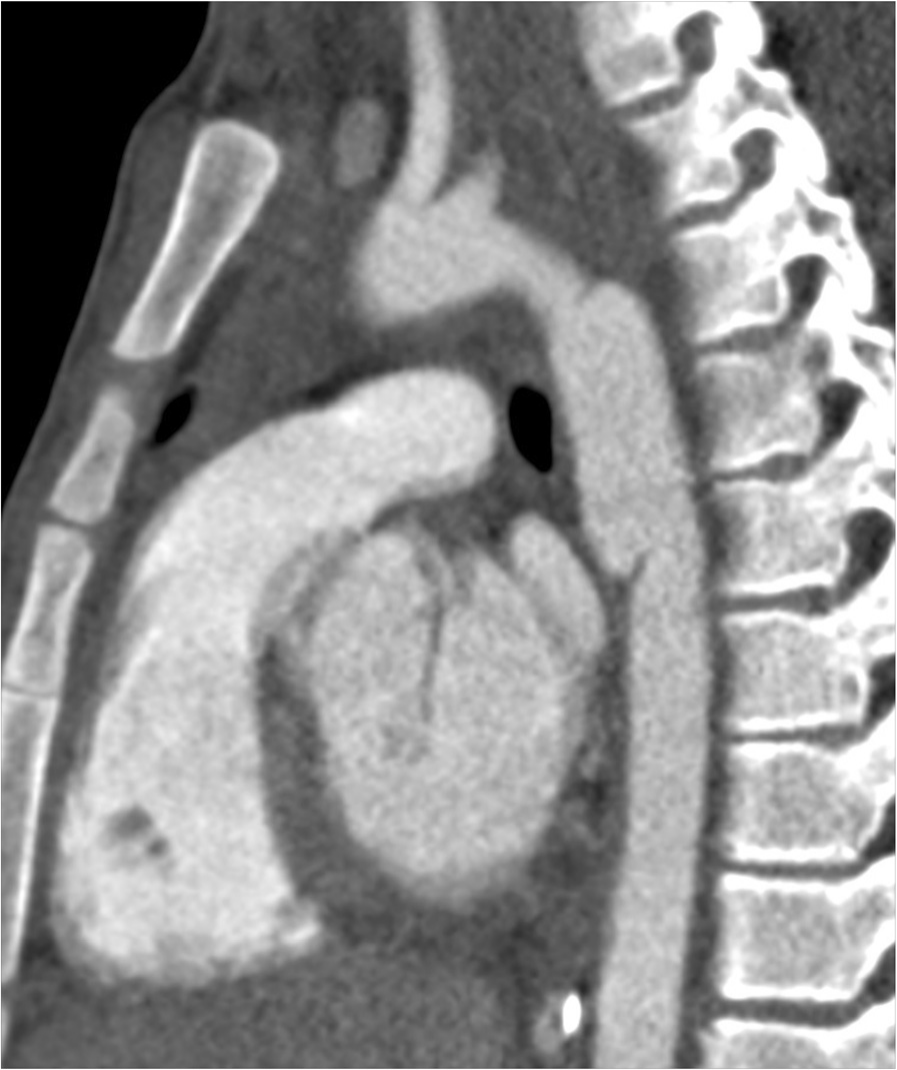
Preoperative CT scan image of traumatic injury to the proximal descending thoracic aorta.
